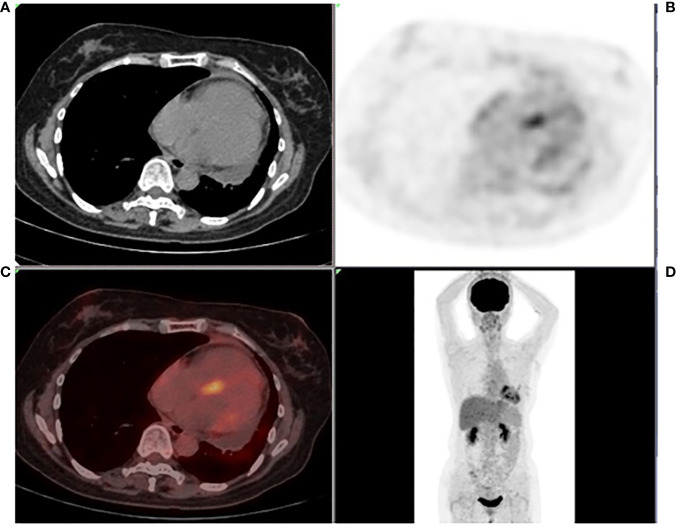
Systemic PET-CT (A–D) showing no clear signs of malignant tumor. The pericardium was slightly thickened and adhered, the metabolism increased slightly, and a small amount of effusions were observed in the pericardium with encapsulated changes.
